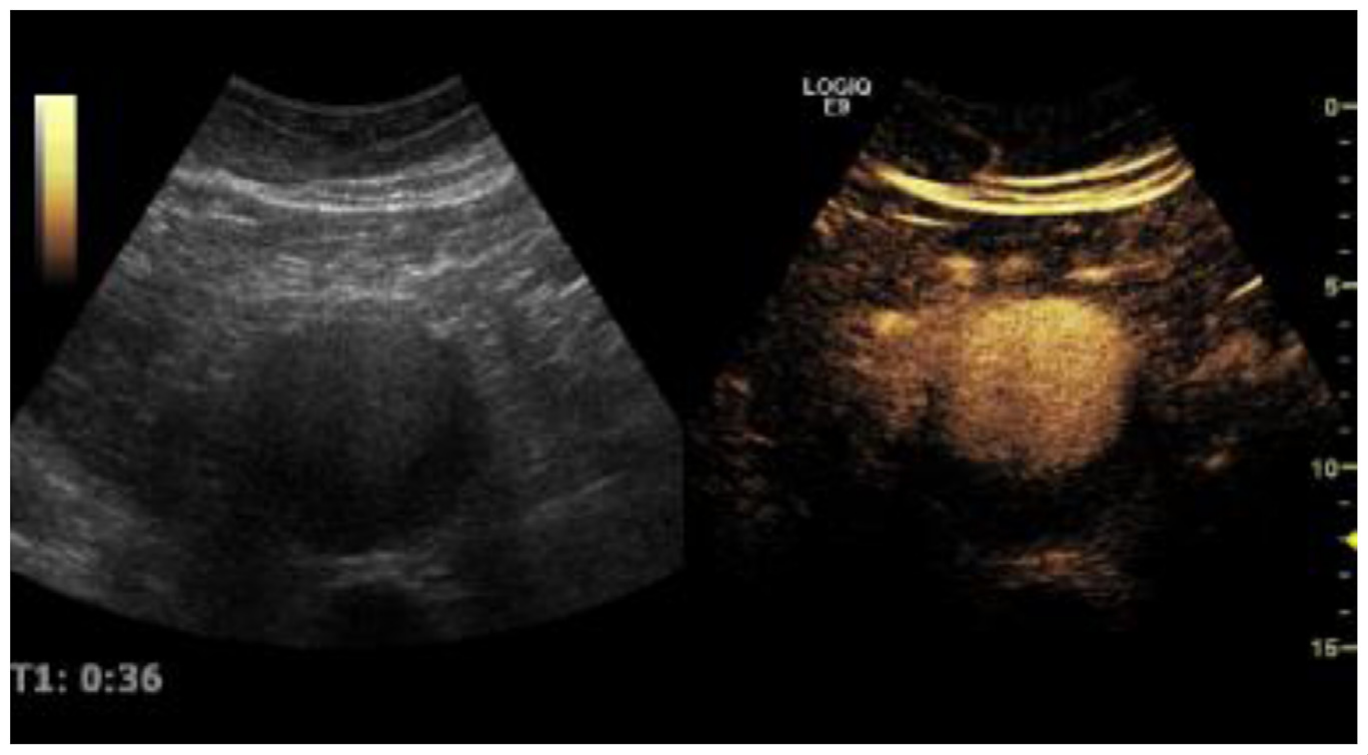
B-mode ultrasound of an abdominal aortic aneurysm (AAA) (*left*). Contrast-enhanced visualization of the AAA prior to microbubble disruption by ultrasound waves (right).
